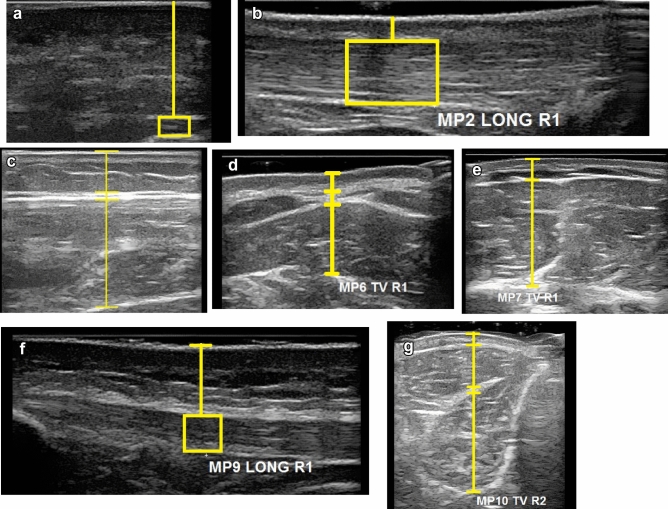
Example of ultrasound, and measurement points for data analysis. (a) Plantar Fascia, (b) Achilles tendon, (c) Soleus, (d) Splenius capitis, (e) Anterior Deltoid, (f) Patellar tendon, (g) Anterior tibialis.
